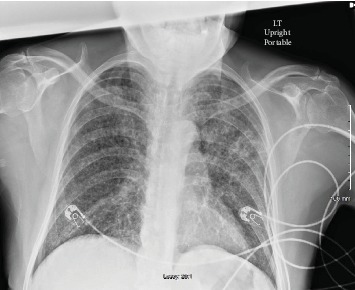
Chest radiograph upon arrival to the emergency department revealed diffuse bilateral reticulonodular opacities and airspace disease with a possible lingular cavitary lesion.
